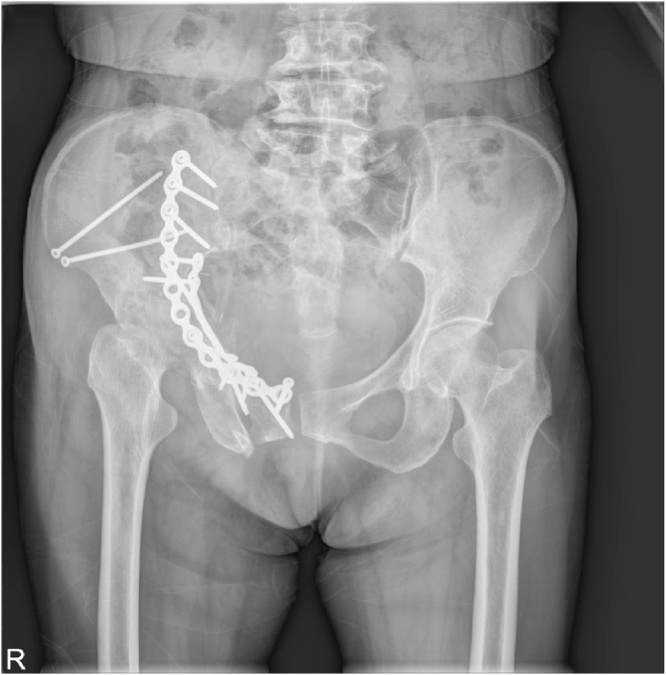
2 months post-operative radiograph of the pelvis showing signs of severe progressive destruction of the right femoral head with joint space narrowing and subchondral bone loss in the femoral head.
